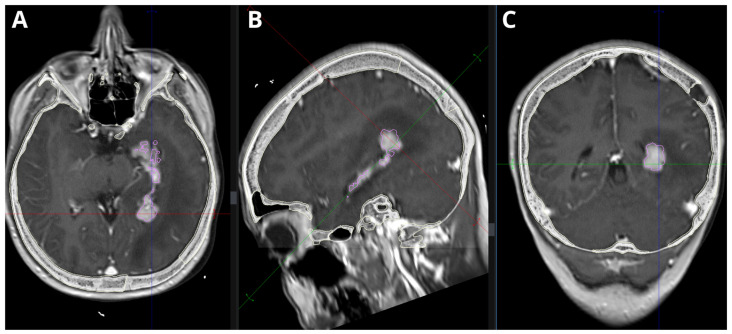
MPR merge CT + FLAIR-weighted MRI showing an intraventricular tumor with extensive edema of the surrounding temporal lobe structures. (A) Axial plane. (B) Saggital plane. (C) Coronal plane.
